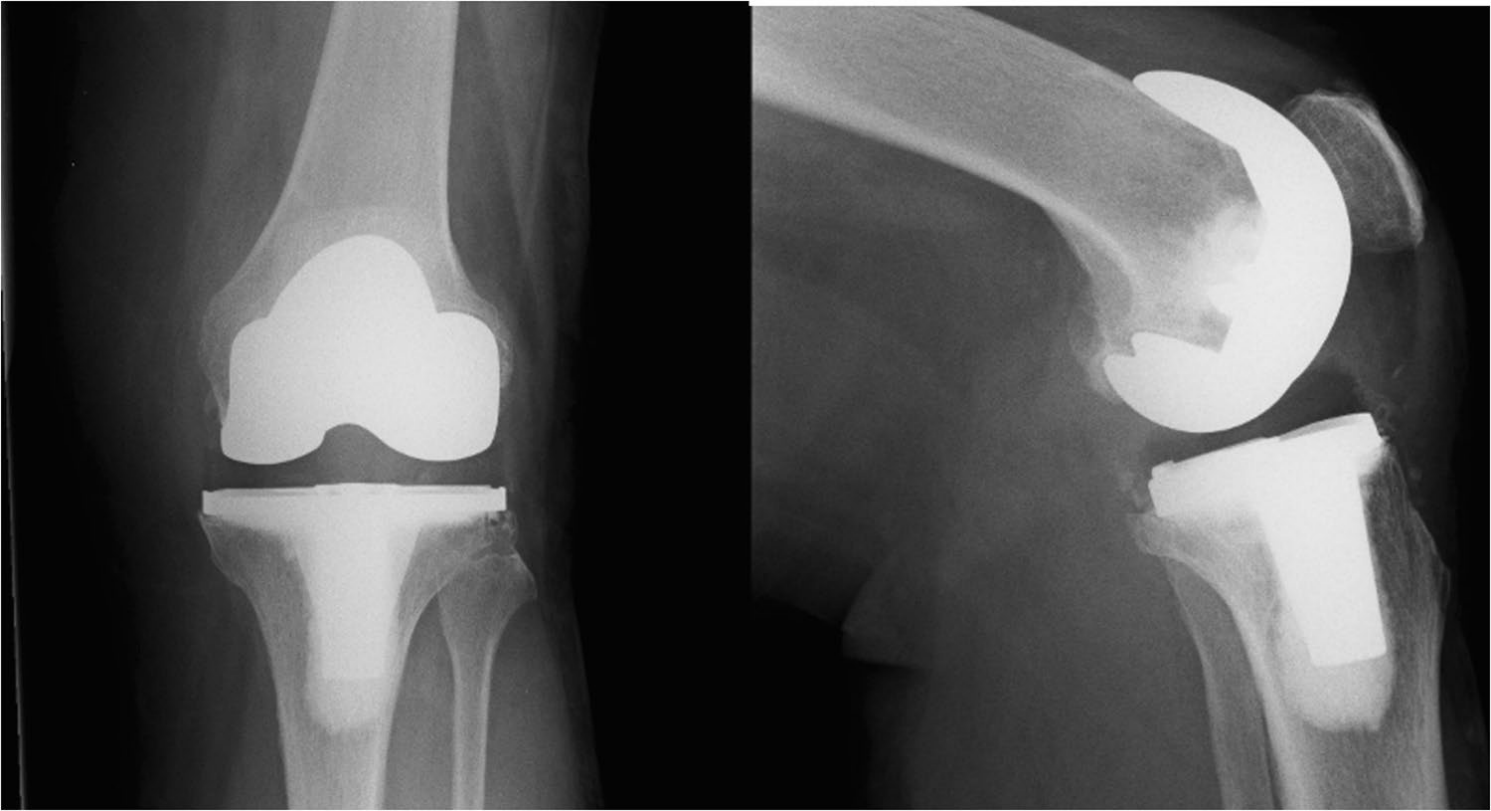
Anteroposterior (AP) and lateral radiographs of a non-augmentable prosthesis. Note the absence of the radiolucent polyethylene plug at the tip of the keel
